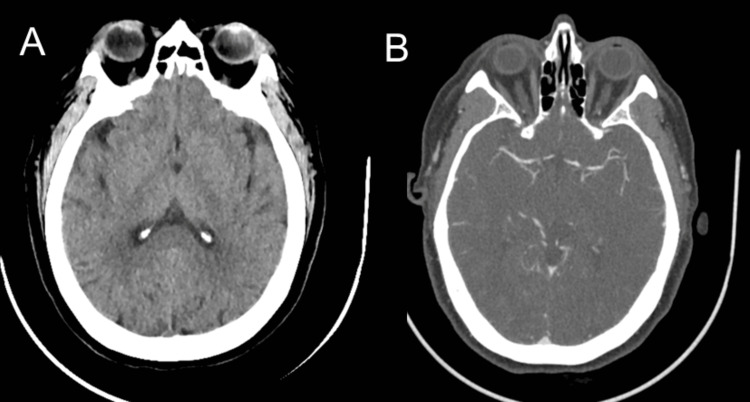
Initial CT and CTA of the head were normal. Computed tomography (CT) (A) and computed tomography angiography (CTA) of the head (B), showing no hemorrhage and no large vessel occlusion (LVO).
